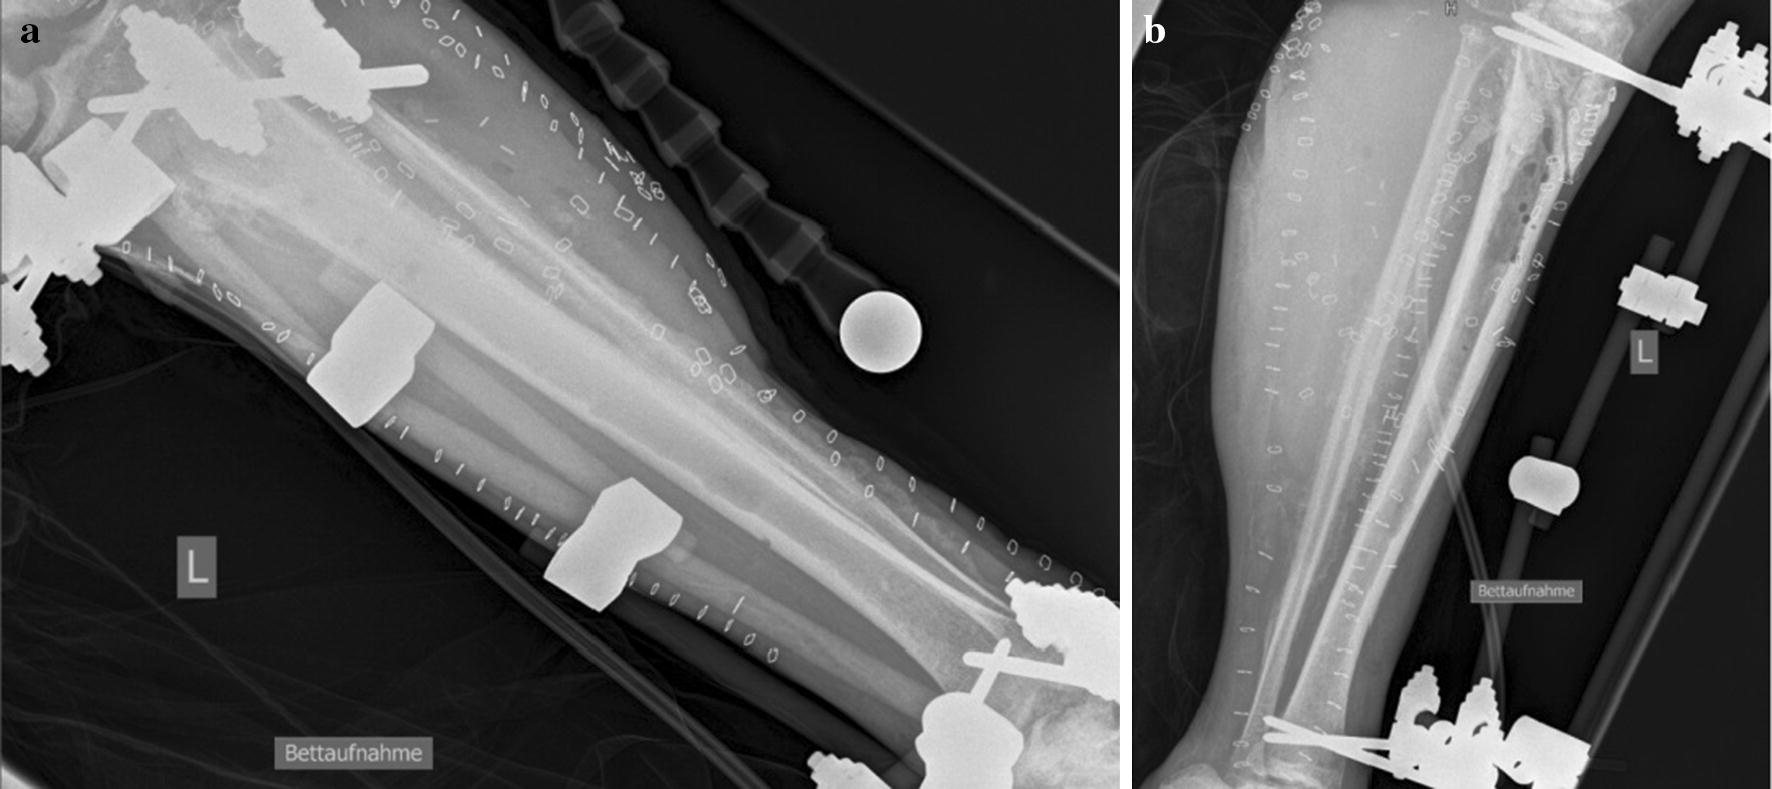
Axial anterior external fixator for securing soft tissue coverage. a X-ray a.p. view and b X-ray lateral view
